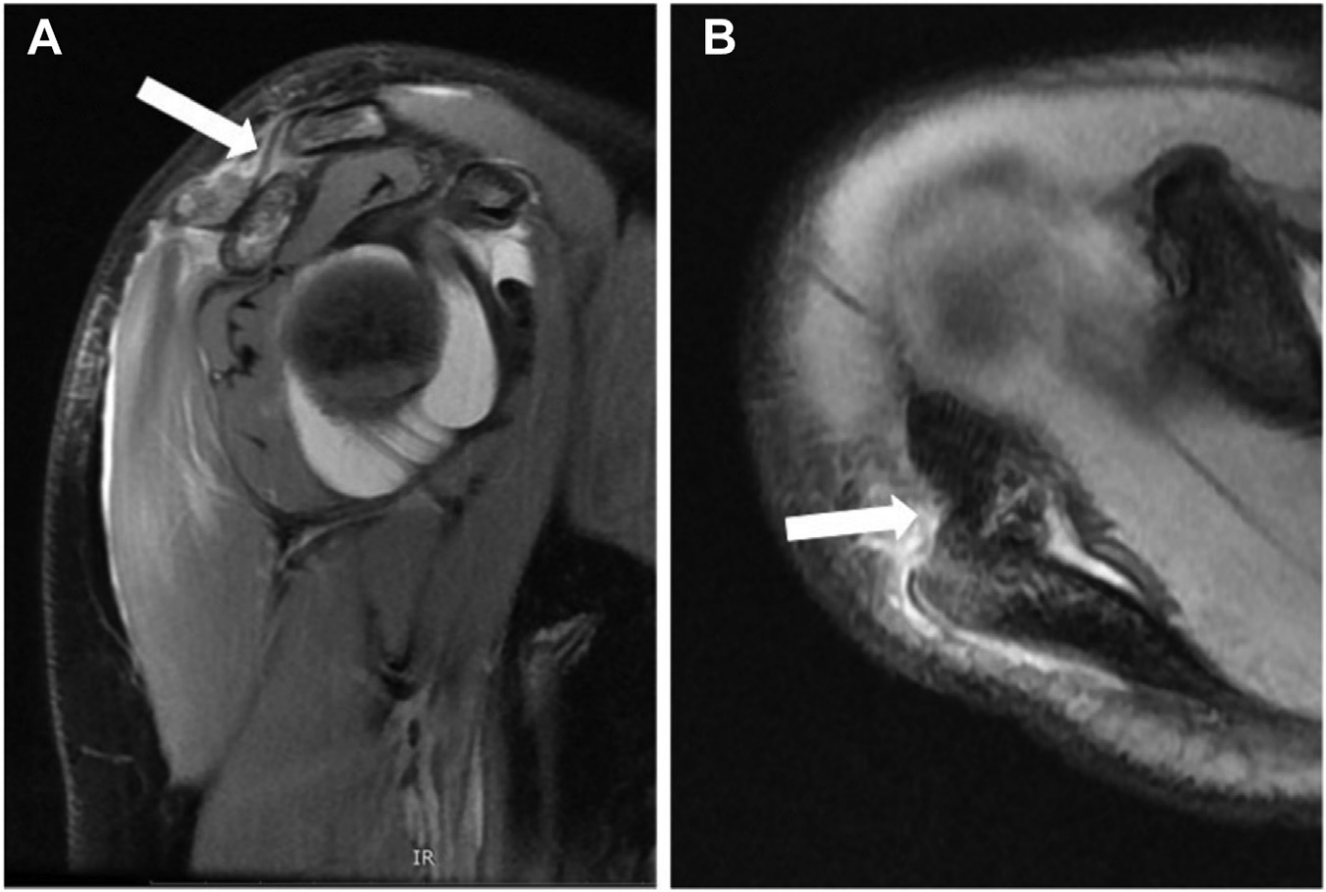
(A) Preoperative sagittal plane MRI showing an inferior displaced type-I Salter-Harris physeal fracture (arrow) with subacromial space occupation. (B) Preoperative axial plane MRI showing anterior displacement of the acromial fragment (arrow). *MRI*, magnetic resonance imaging.
